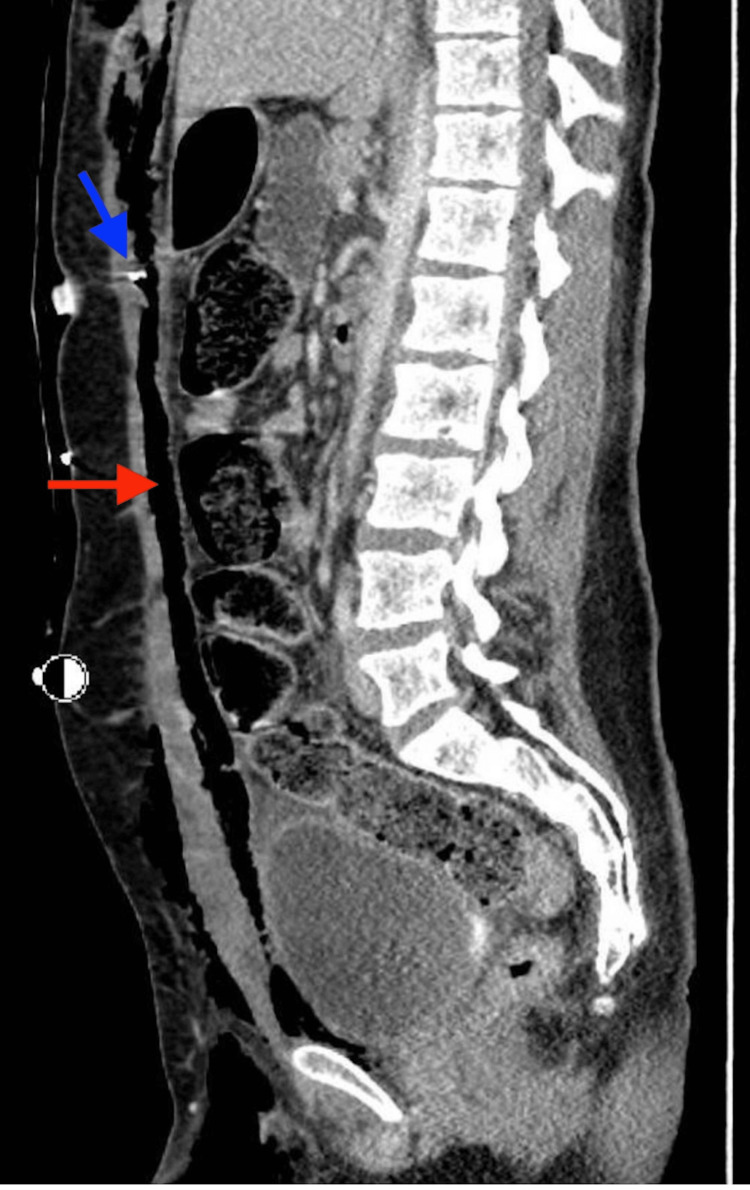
Sagittal CT of the chest, abdomen, and pelvis with intravenous contrast. Vertical tracking of extraluminal air and enteric contents anterior to the peritoneum from the malpositioned gastrostomy tube site. The blue arrow indicates the malpositioned gastrostomy tube in the retrorectus space. The red arrow indicates the extraluminal air and enteric contents anterior to the peritoneum.
